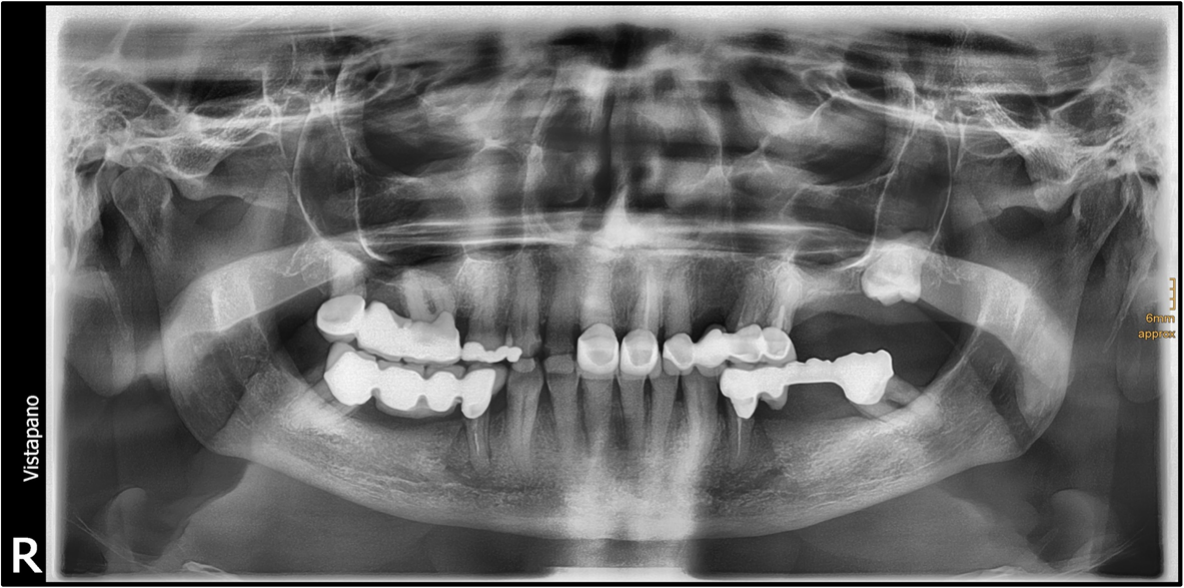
Panoramic radiograph before tooth extraction
